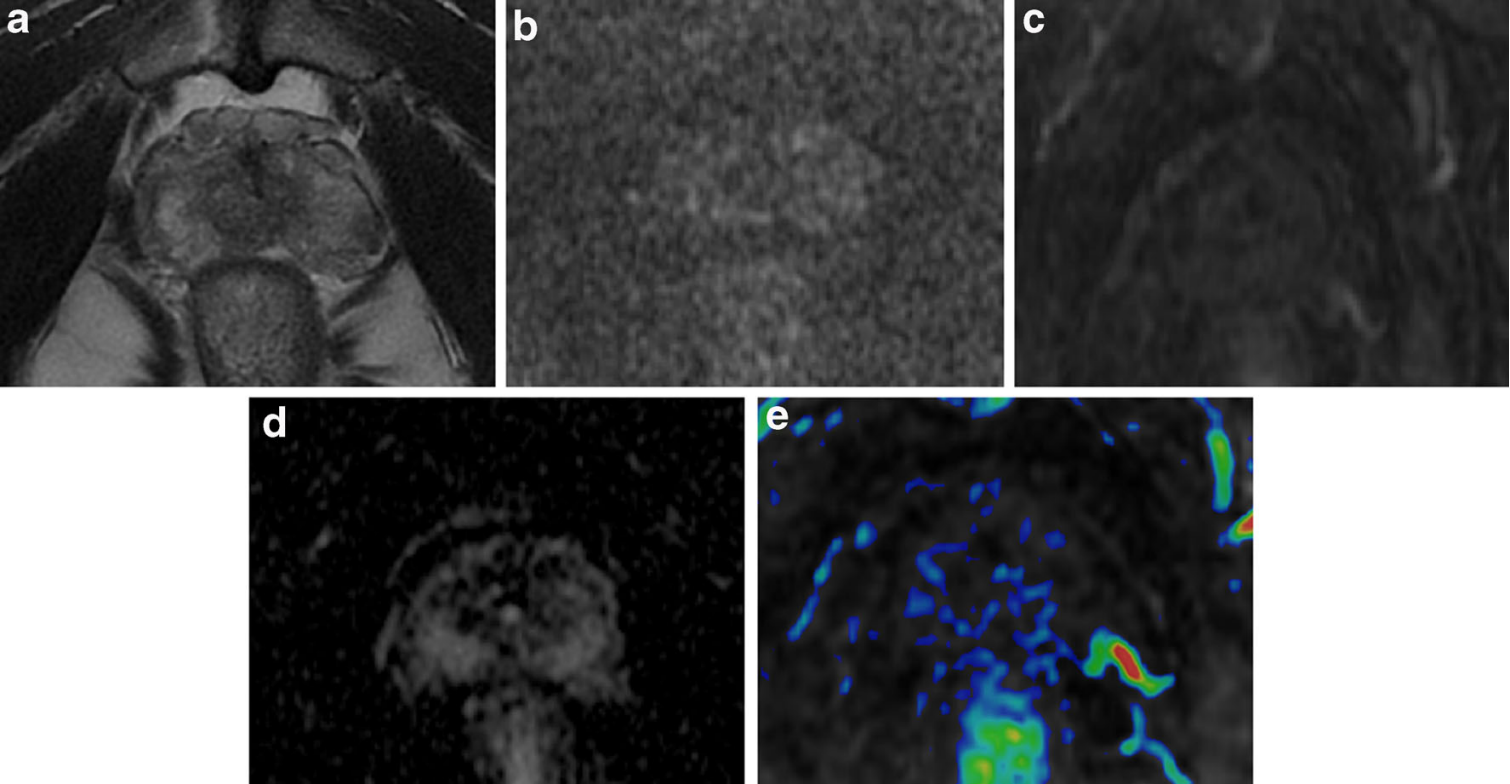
Prominent peri-prostatic bundle with no ERC. a Axial T2-weighted fast spin-echo image showing an ill-defined hypointense area in posterior left peripheral zone, suspected for cancer. b Axial DWI image with b value = 3000 mm^2^/sec, and c ADC map image showing no areas of significant restriction of water diffusivity. d Axial perfusion gradient-echo T1-weighted image, and e colour DCE MR map showing no enhancing areas in left peripheral zone with and enhancing neuro-vascular bundles adherent to the glandular profile. According to he PIRADSv2 scoring system, the score for T2, DCE and DWI is, respectively: 2, + and 4; the overall score is 4 (based on dominant sequence), which means that the presence of clinically significant cancer is equivocal
